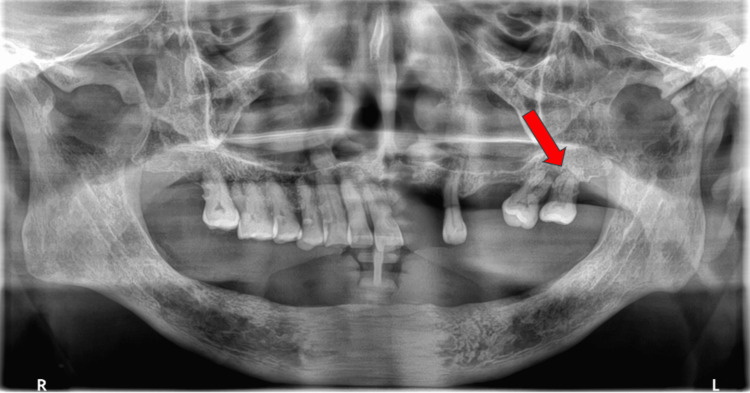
OPG showing left upper third molar with apical bone loss (red arrow) Apical bone loss, a feature of severe periodontitis, involving tooth 28, was observed along with loss of adjacent teeth. OPG, orthopantomogram
